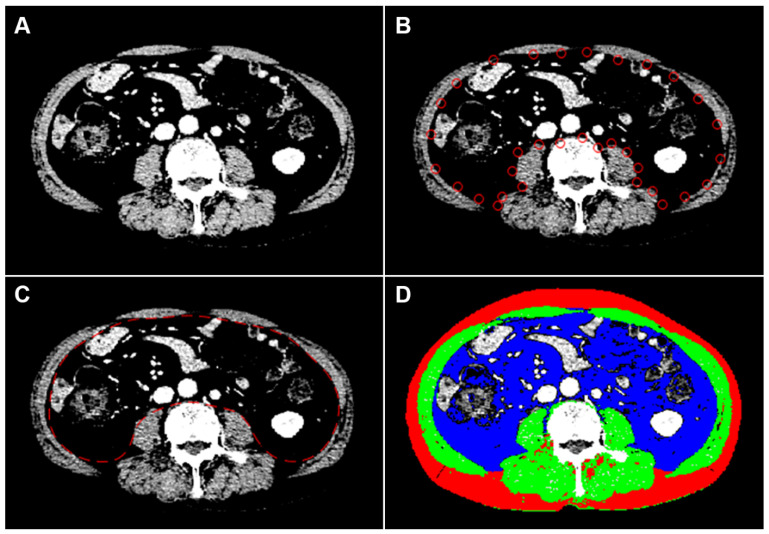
An example of semiautomatic quantification of body composition in a 75-year-old man with metastatic hormone-sensitive prostate cancer. To highlight the muscle boundary, the intensity of the CT image is linearly transformed into 0 to +100 HU (A). After semiautomatic manipulation (B), the boundary between the muscles and the inner tissues is detected using the active contour method by minimizing a cost function, dividing CT images into inner and outer regions (C). Pixels in the fat and muscle are then identified using cut-off values of −300 to −50 HU and −29 to +150 HU, respectively (D). The cross-sectional areas of muscle, subcutaneous fat, and visceral fat are measured to be 140.03 cm^2^, 118.69 cm^2^, and 181.37 cm^2^, respectively. Green-colored, red-colored, and blue-colored areas represent muscle, subcutaneous fat, and visceral fat, respectively; CT, computed tomography; HU, Hounsfield unit.
